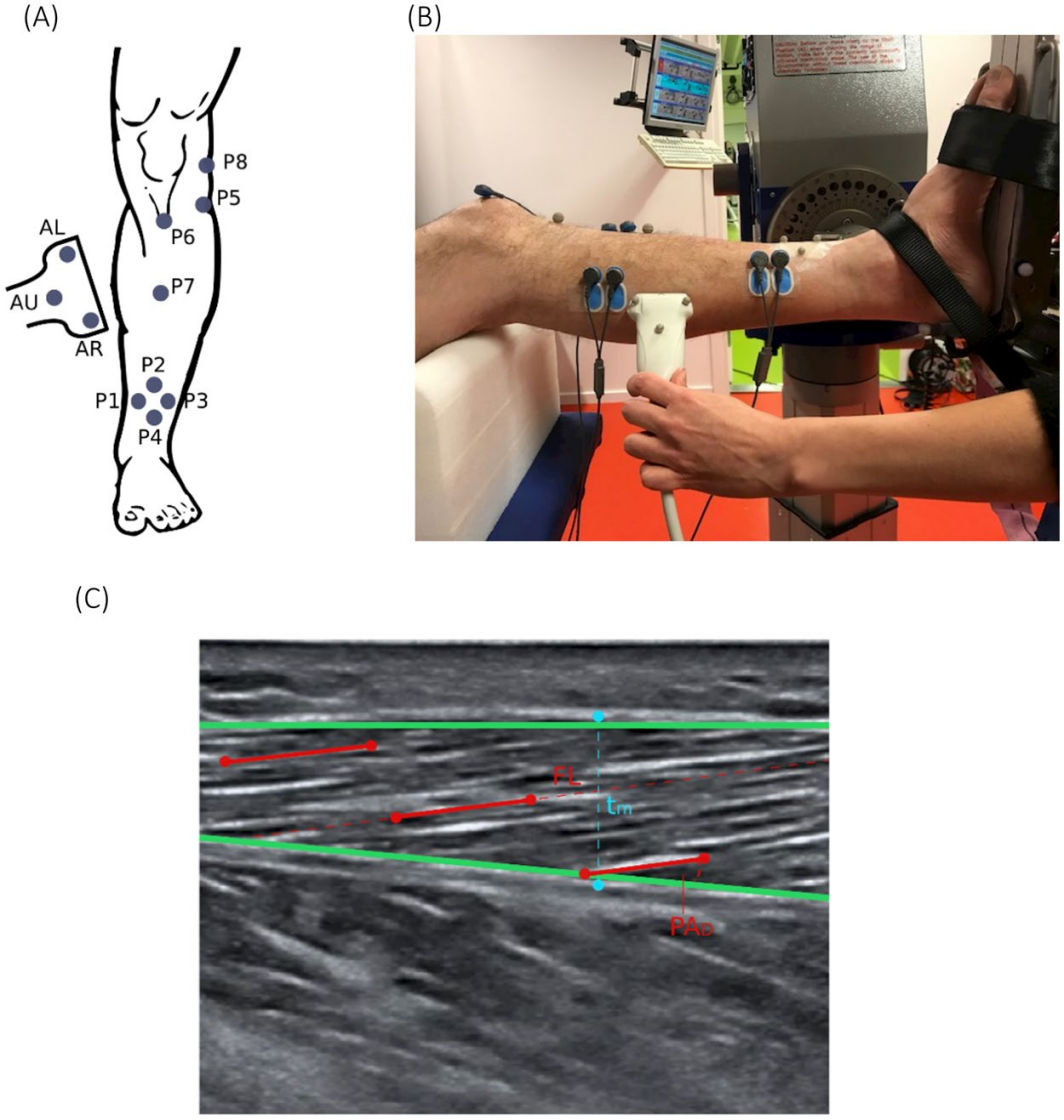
(A,B) Marker placement and experimental set-up during the ultrasound measurement. Markers P1, P2, P3 and P4 were fixed on to a rectangle silicon pad and attached to the lower limb. Marker P5 and P6 were placed on the head of the fibula and the tuberosity of the tibia, respectively. Marker P7 was placed 10 cm distal to P6 along the tibia bone. P8 was placed on the medial side of the epicondyle. Marker P4, P7 and P6 were carefully adjusted in order to align in a straight line. AL, AR and AU were the markers placed on the US transducer, where AL and AR defined the acquisition window of the transducer. (C) Example of the measurement of the parameters pennation angle (PA), muscle thickness (t_m_) and fascicle length (FL) of the tibialis anterior muscle in an US image using MATLAB (green lines: deep and superficial aponeurosis, red lines: selected fascicles). The muscle thickness was identified by setting two points (blue markers) marking each of the aponeuroses.
